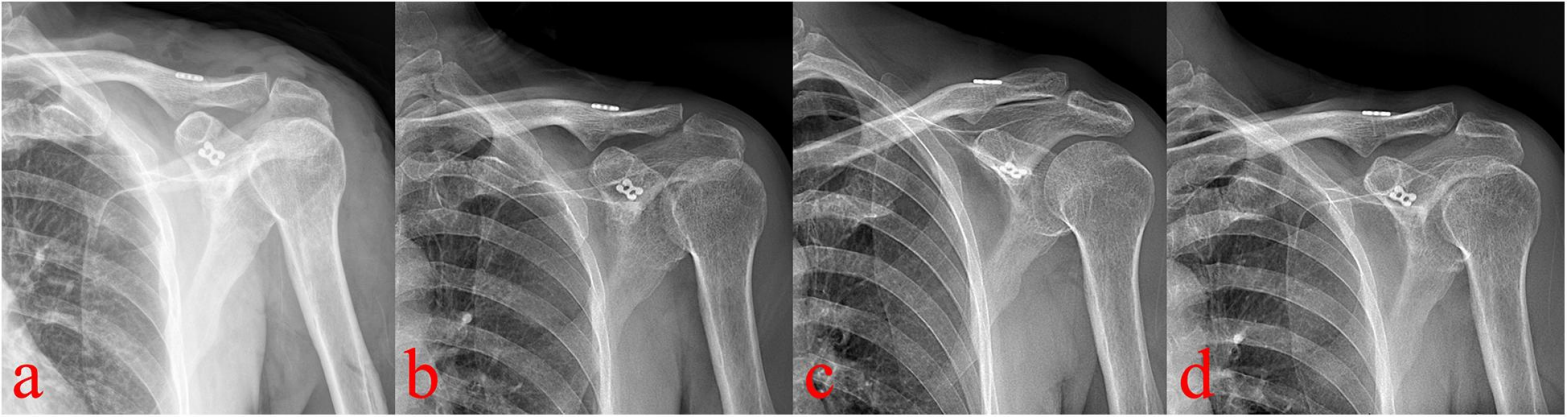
Postoperative radiographs (a–d, immediate postoperative, 3-month, 6-month, and 1-year) show progressive clavicular tunnel enlargement until 6 months, then stabilization, with no coracoid tunnel widening. A minor loss of AC joint reduction observed.
